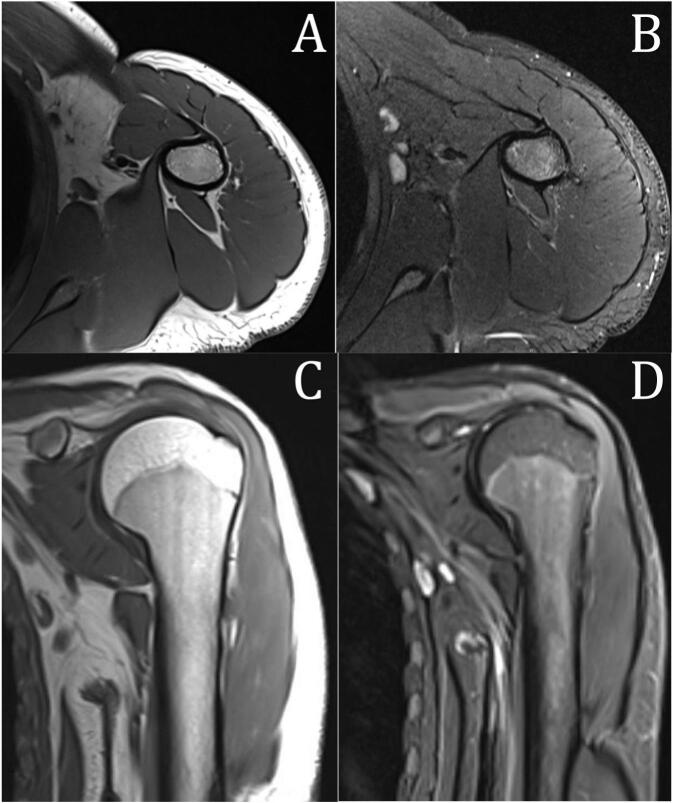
MRI of the left proximal humerus, A & B: T1 & T2 fat sat axial cuts showing almost complete resorption of the osteochondroma with only bony remnant at the posterolateral aspect of the proximal third of the humerus. C & D: T1 & T2 fat sat coronal cuts showing almost complete resorption of the lesion.
